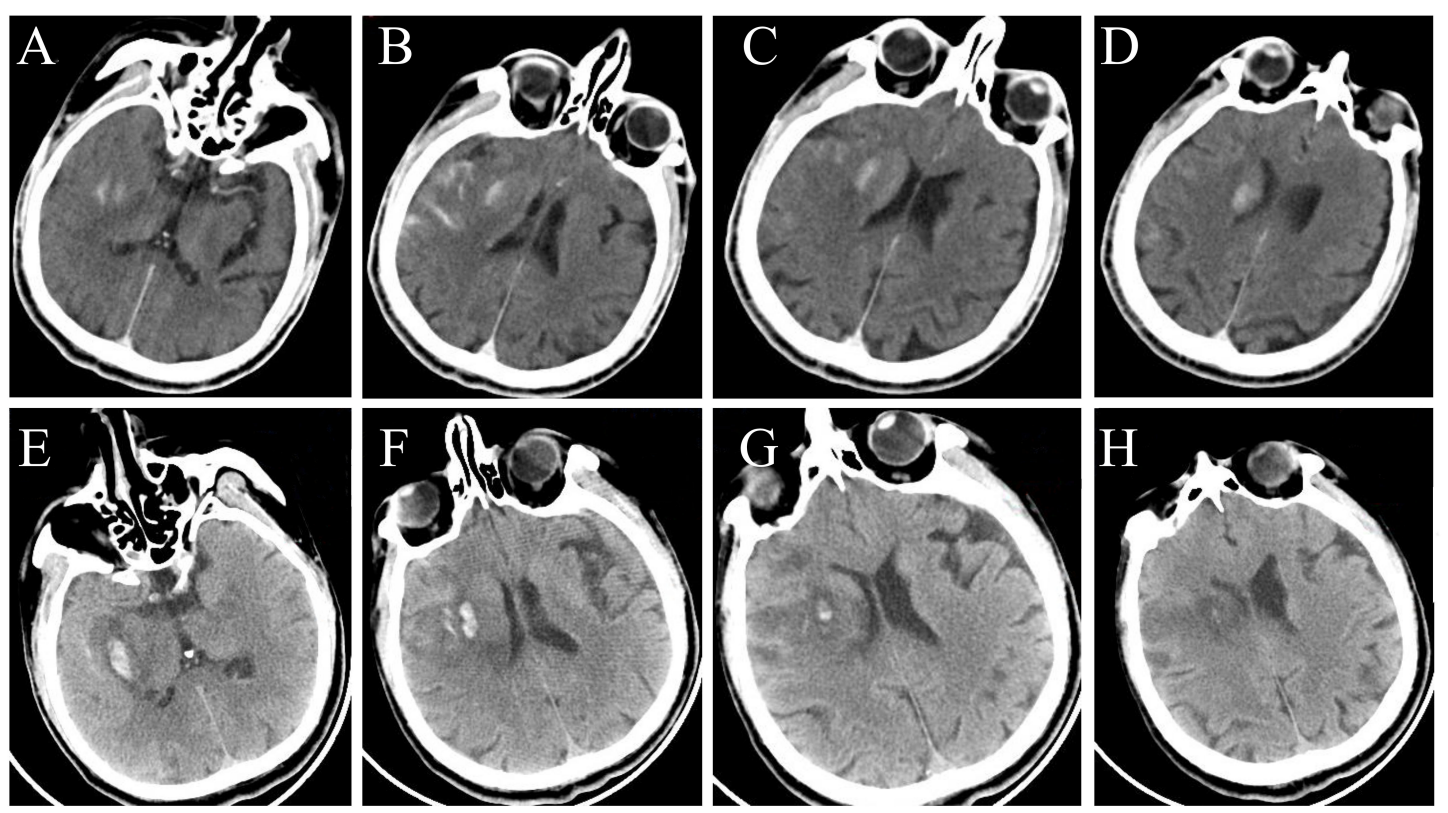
Representative images of parenchymal hyperdensity immediately after thrombectomy on NCCT (A–D) for Post-ASPECTS assessment; and NCCT images (E–H) at 5 days after thrombectomy showing HT. NCCT, non-contrast computed tomography; Post-ASPECTS, post-thrombectomy ASPECTS; HT, hemorrhagic transformation.
